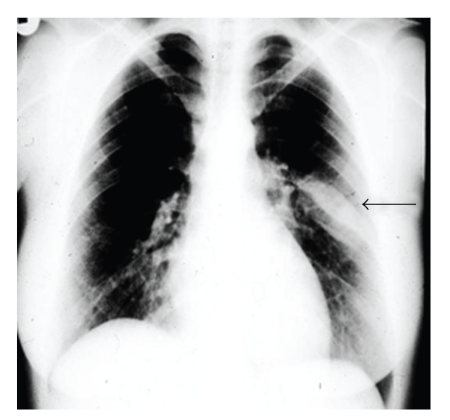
Chest X-ray shows a lesion in the 7th left rib.
